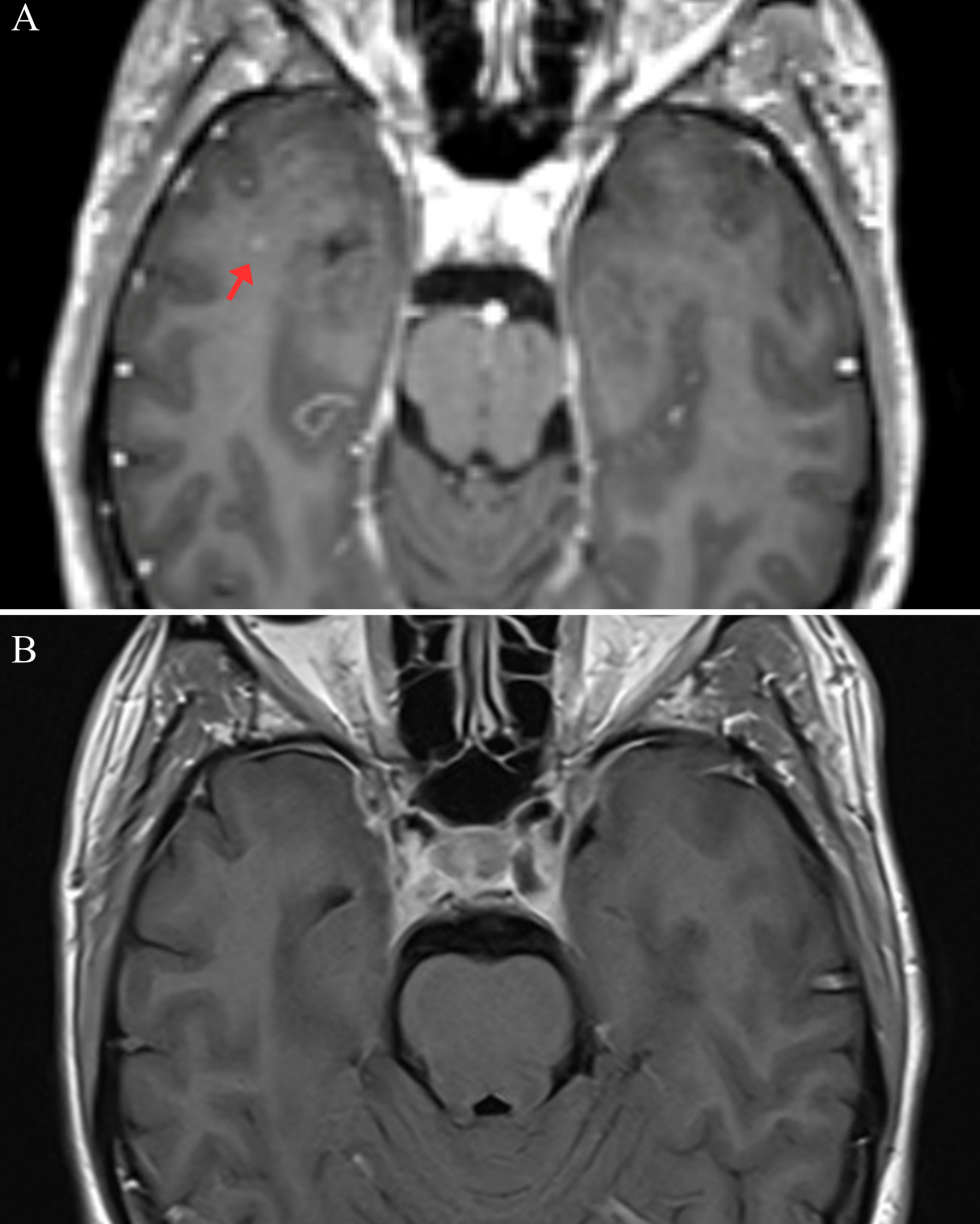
The evolution of contrast-enhancing lesion. (A) Axial post-contrast T1 at first magnetic resonance imaging (MRI) clearly shows the small, intensely enhancing lesion in the right temporal lobe (red arrow) prior to treatment. (B) Axial post-contrast T1 on the second MRI, day five post-corticosteroid administration, demonstrates a complete resolution of contrast enhancement in the previously enhancing right temporal lesion, which supports a steroid-responsive inflammatory pathology rather than a vascular process.
